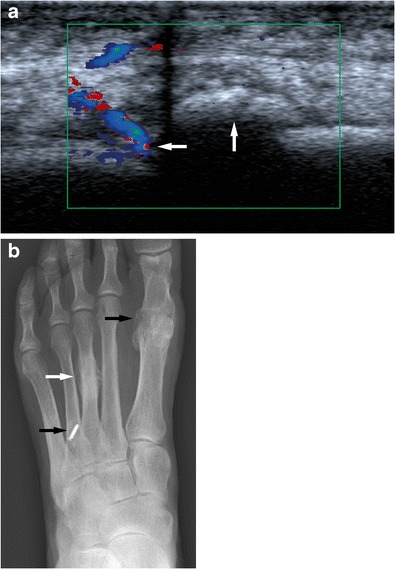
An elderly female attending for an ultrasound of the foot to assess forefoot pain. A longitudinal ultrasound image (left side is proximal) demonstrates partly ossified hypervascular callus (white arrows) of a metatarsal (a) suggestive of a healing metatarsal fracture. A PA radiograph of the left foot (b) shows evidence of previous surgery (white arrows) and a typical stress fracture of the left third metatarsal (white arrow). Previous surgery particularly of the feet is a risk factor for the development of stress fractures. Ultrasound is not the imaging modality of choice for the assessment of stress fractures but can incidentally show stress fractures
